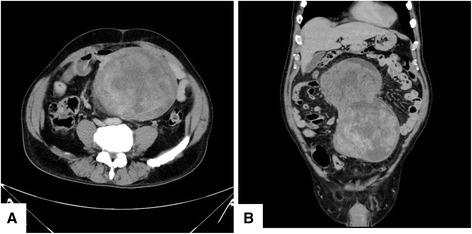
(A) Abdominal computed tomography demonstrated a 25 × 11 cm, heterogeneous, lobulated mass in the abdominal cavity. (B) Colonal view demonstrated lobulated mass.
